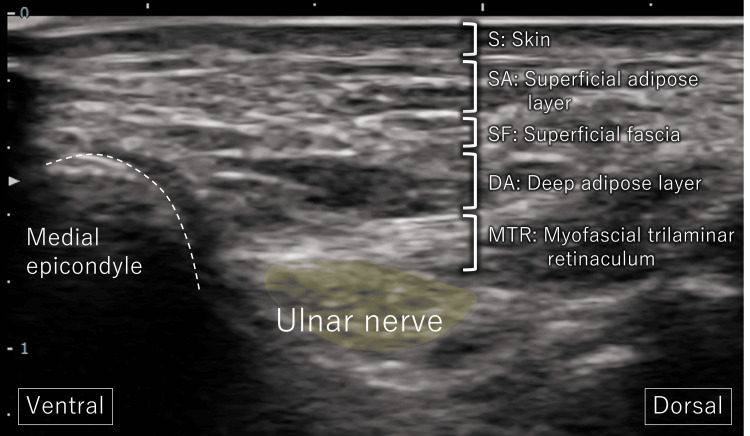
Anatomical depiction of the UN at the cubital tunnel Ultrasound depiction of perineural structures surrounding the UN, including the MTR. UN: ulnar nerve, MTR: myofascial trilaminar retinaculum, S: skin, SA: superficial adipose layer, SF: superficial fascia, DA: deep adipose layer
